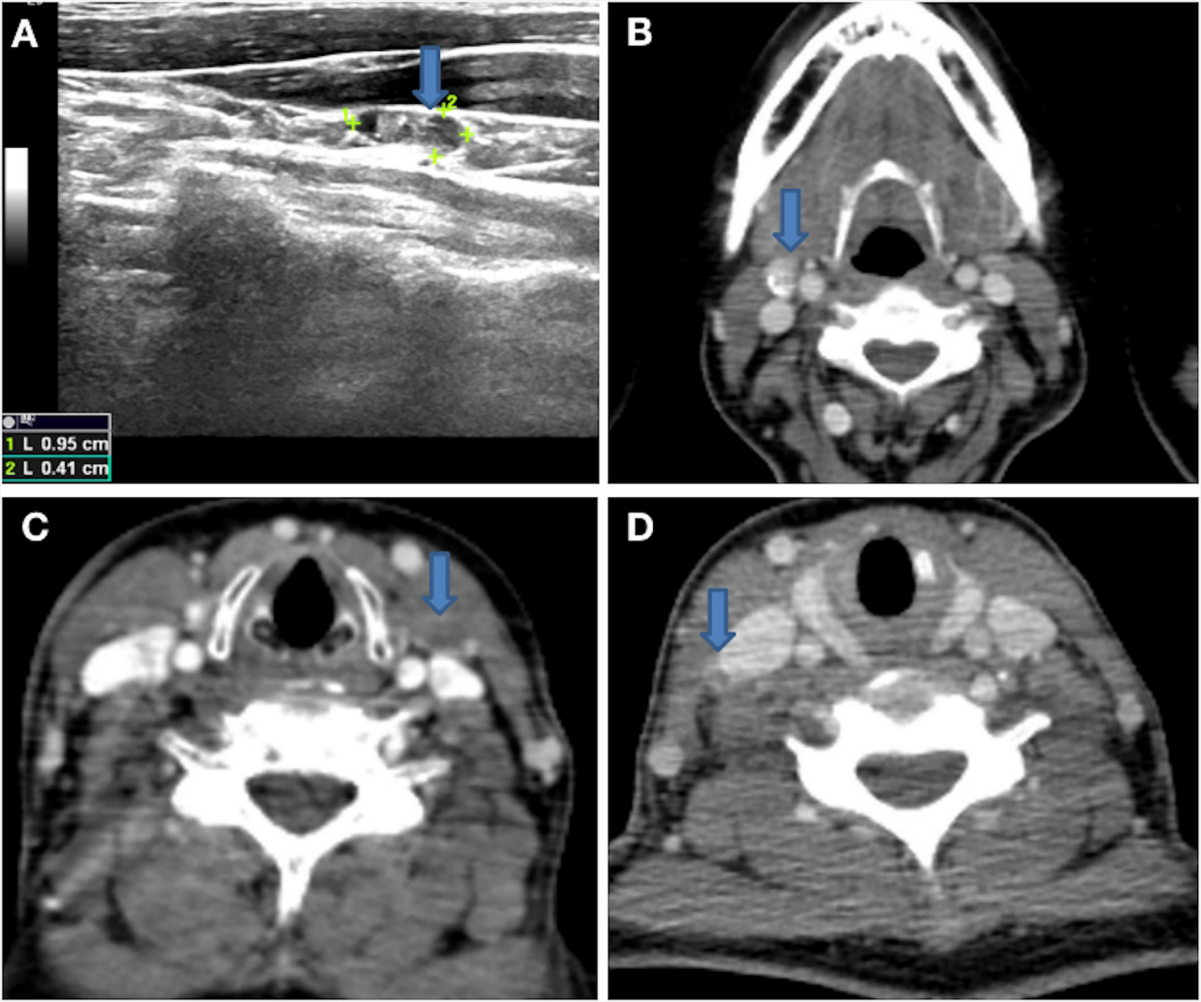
(A) Lateral neck lymph node with microcalcification on high-resolution US. (B) Lateral neck lymph node with microcalcification on 1-mm thin layer contrast-enhanced CT. (C, D) Lateral neck lymph nodes with low-density or liquid components on 1-mm thin layer contrast-enhanced CT.
